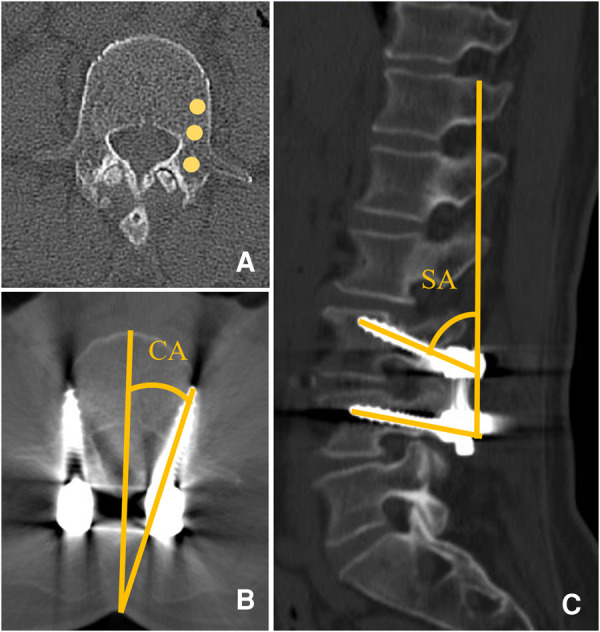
(A) HU measurement of an average of three points identified according to the screw track in the preoperative lumbar CT scan. (B) CA was defined as the angle between the screw and spinous process in the axial plane. (C) SA was defined as the angle between the screw line and vertical line in the sagittal plane.
